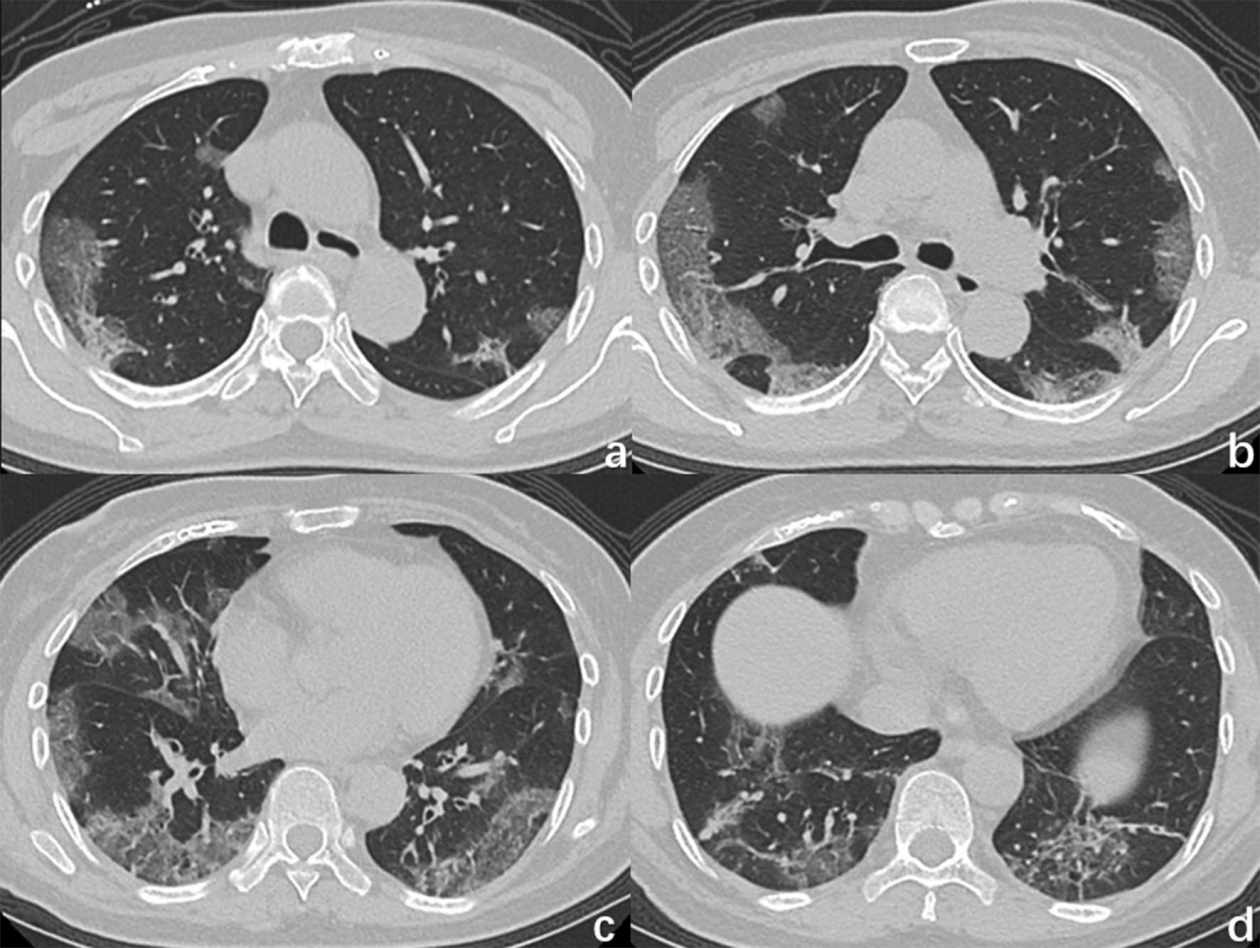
CT image of a 66-year-old man with COVID-19 infection, presenting fever with cough for 7 days, shows multiple GGOs in bilateral lung, mainly distributedin the subpleural areas, with reticular pattern (interlobular septal thickening).
